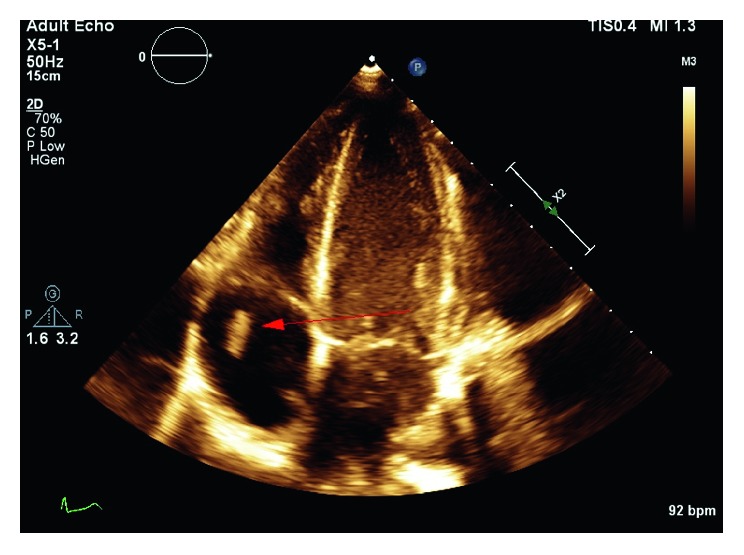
Preprocedural transthoracic echocardiogram (TTE). Apical 4-chamber view revealing a 3 × 1.5 cm tricuspid valve vegetation (red arrow).
